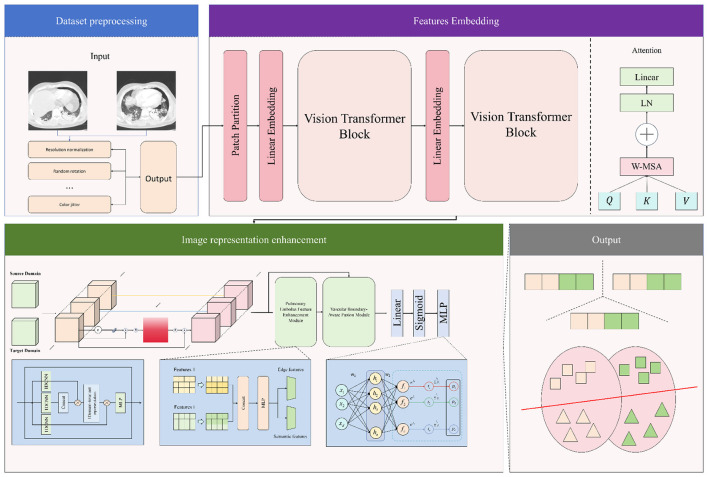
The overall framework of the proposed method for pulmonary embolism recognition from CT images. The model consists of dataset preprocessing, feature embedding, image representation enhancement, and output prediction, where the Pulmonary Embolus Feature Enhancement Module and the Vascular Boundary-Aware Fusion Module are introduced to strengthen lesion-related discriminative representations and vascular boundary-aware semantic modeling.
